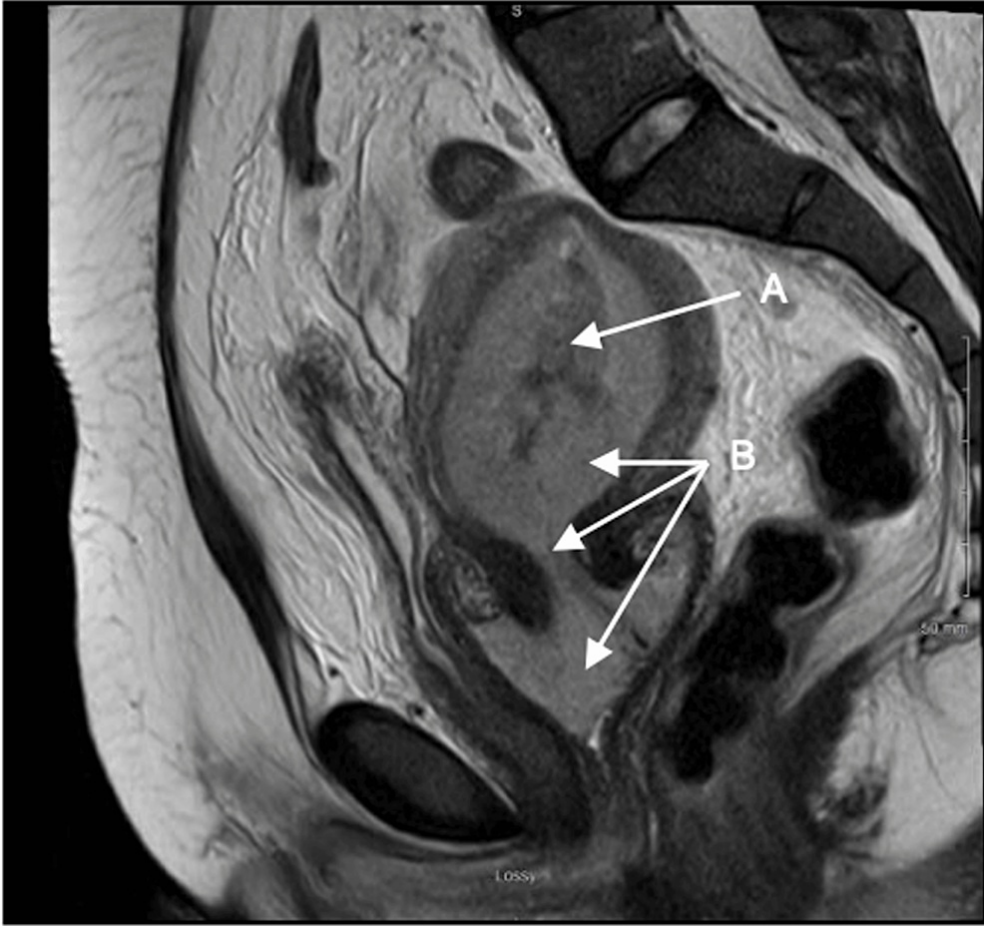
MRI of the pelvis, sagittal view. MRI of the pelvis demonstrates a (A, arrow) heterogeneous T2 hypointense region in the endometrium measuring 2.2 cm in thickness with areas of T2 hypointensity. (B, arrow) Intermediate T2 hyperintense sub-endometrial soft tissue surrounding the endometrial stripe, extending into the endocervical canal, and distending the vaginal cavity. MRI: magnetic resonance imaging
